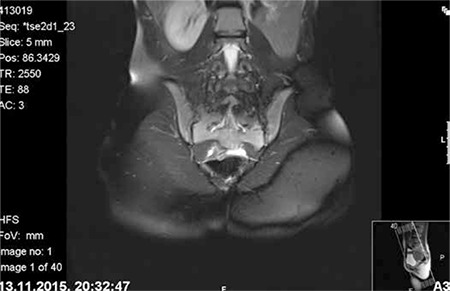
Magnetic resonance image demonstrating left renal agenesis
